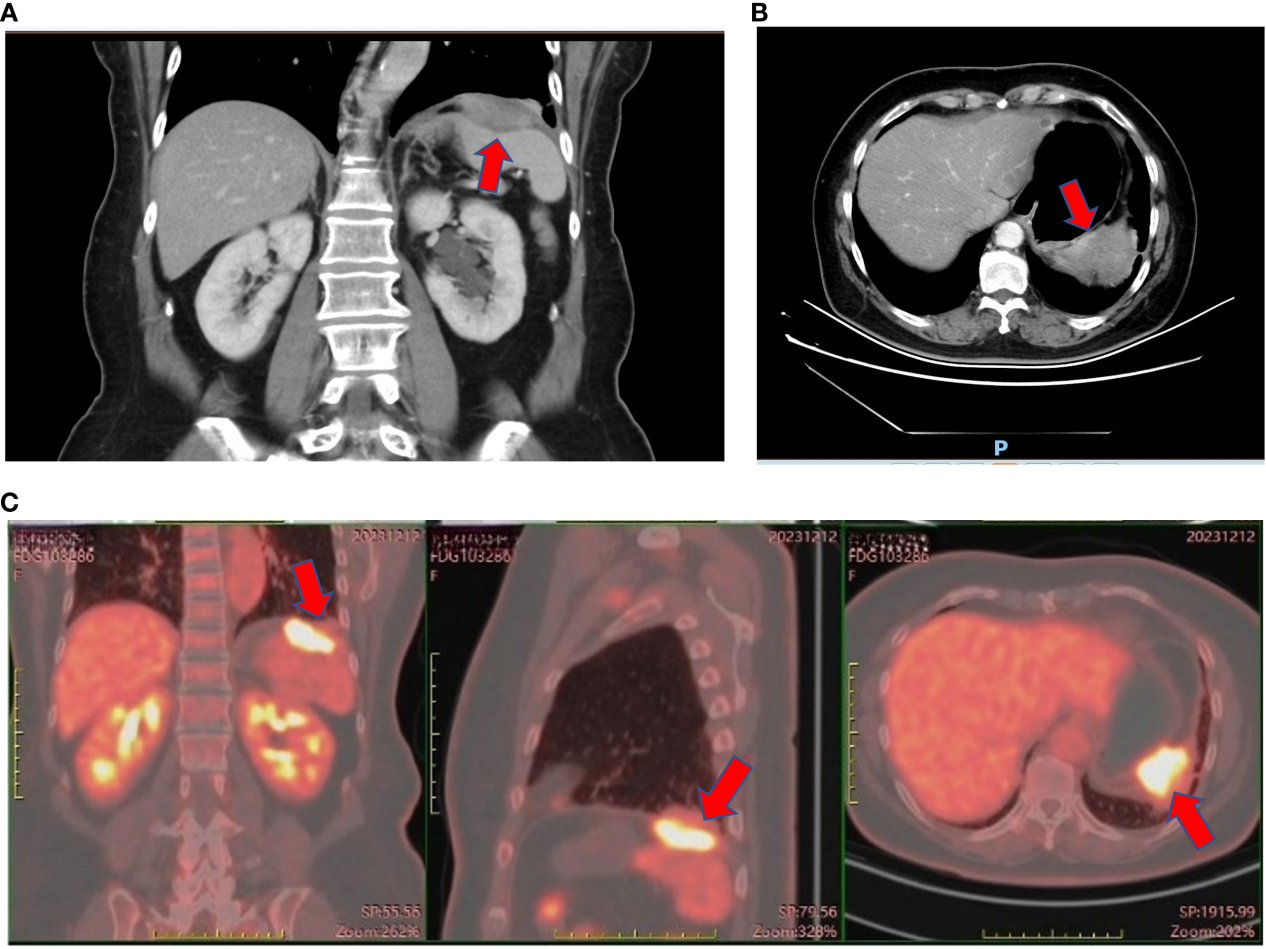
Enhanced CT and PET-CT of the left subdiaphragmatic mass. (A) Coronal view of enhanced CT of the left subdiaphragmatic mass on November 14^th^, 2023. (B) Axial view of enhanced CT of the left subdiaphragmatic mass on November 14^th^, 2023. (C) PET-CT of the left subdiaphragmatic mass on December 12^th^, 2023.
